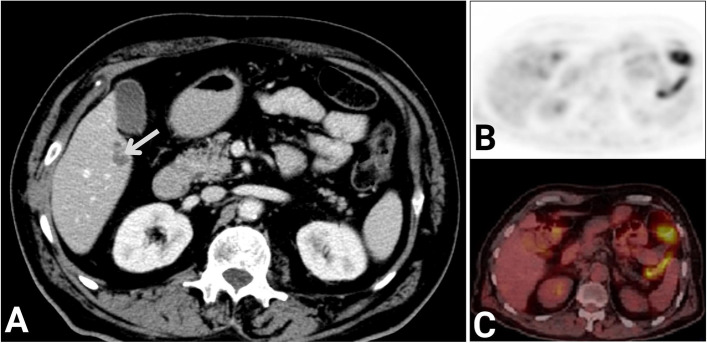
A 60-year-old patient diagnosed with bladder cancer who underwent ^18^F-FDG PET/CT for staging. Diagnostic contrasted CT (a) showed a suspicious liver lesion in segment V (white arrow) that was referred for further evaluation. Axial PET (b) and axial PET/CT images (c) did not demonstrate any increased metabolic activity in this lesion suggesting being benign in nature. This which was later proven on follow-up
